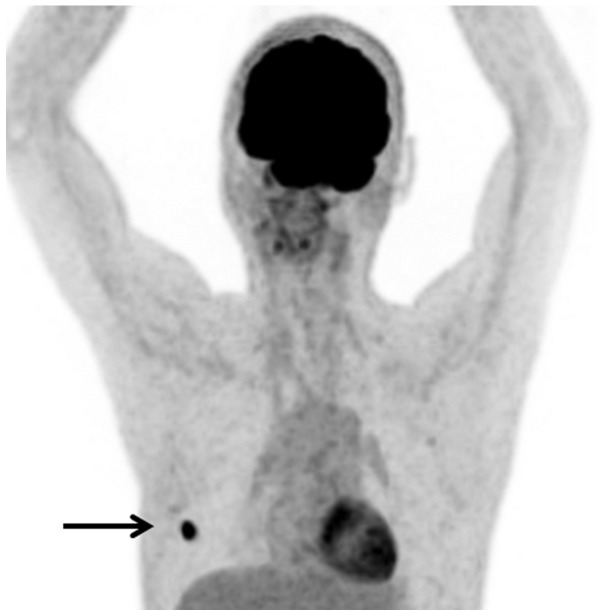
Case two: Positron emission tomography-computed tomography revealing rib metastasis (arrow).
